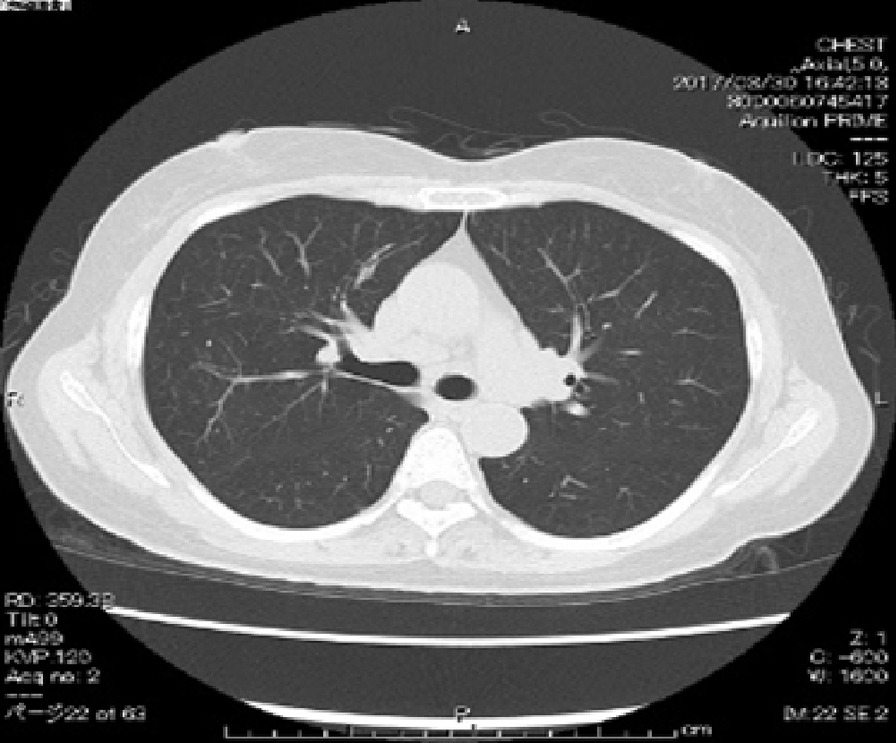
Chest computed tomography. Hilar and mediastinal lymph node swelling and numerous nodular lesions are seen in the bilateral middle lung lobes
